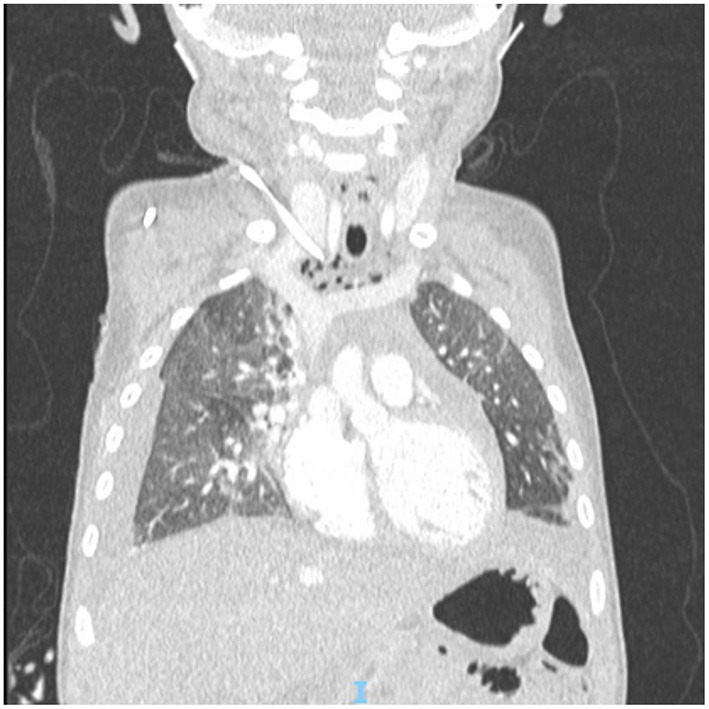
CT scan showing dislocated tip of the catheter, pneumomediastinum and bilateral hydrothorax.
